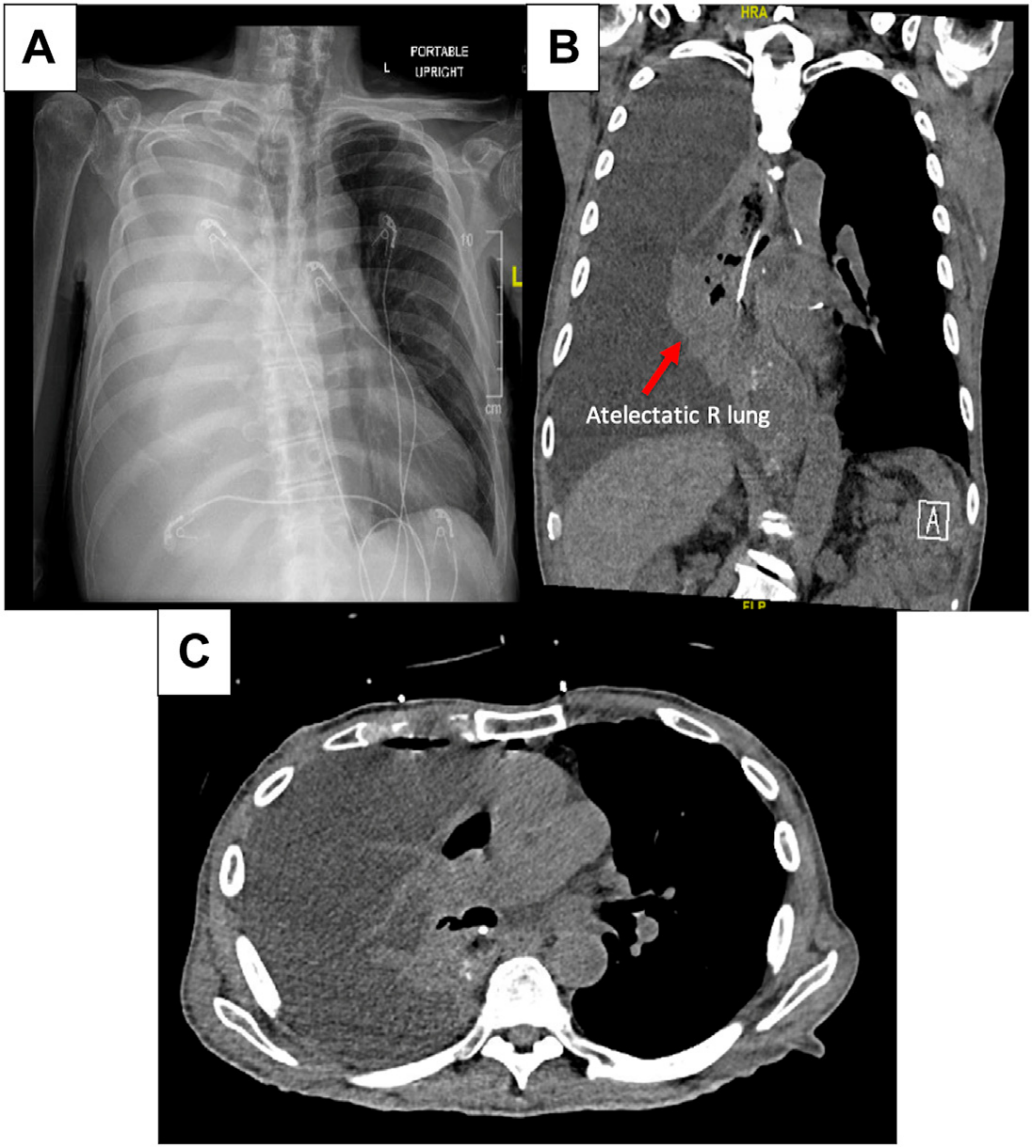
Initial chest imaging on presentation to the emergency department. (A) Chest film shows complete hemi-opacification of the right side of the chest. (B) Coronal computed tomography image shows nearly complete atelectasis of the right lung (arrow). (C) Axial computed tomography image shows right-sided hydropneumothorax. R, right.
